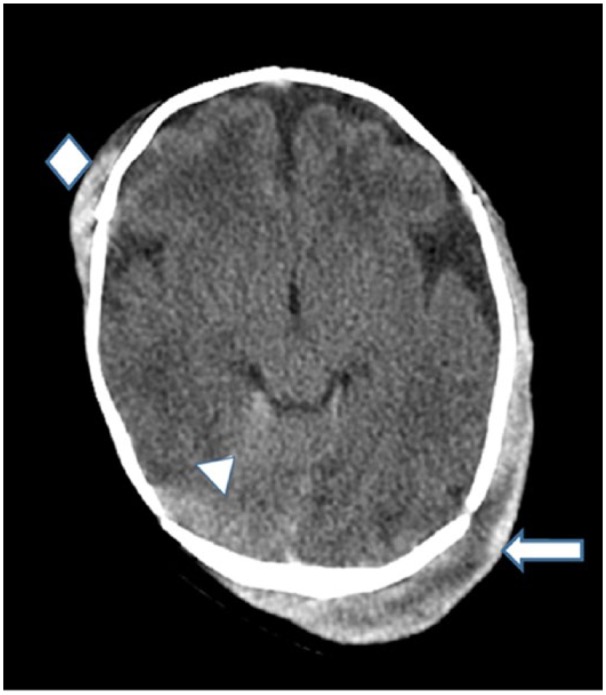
Computed tomography of the head without contrast of infant in case 3. A subdural bleed is seen along the tentorium (arrowhead). A subgaleal hematoma measuring 1.2 cm in thickness involves the left frontoparietal region (arrow). Another subgaleal hematoma measuring 5 mm in thickness is seen in the right frontoparietal region (diamond). No evidence of midline shift.
